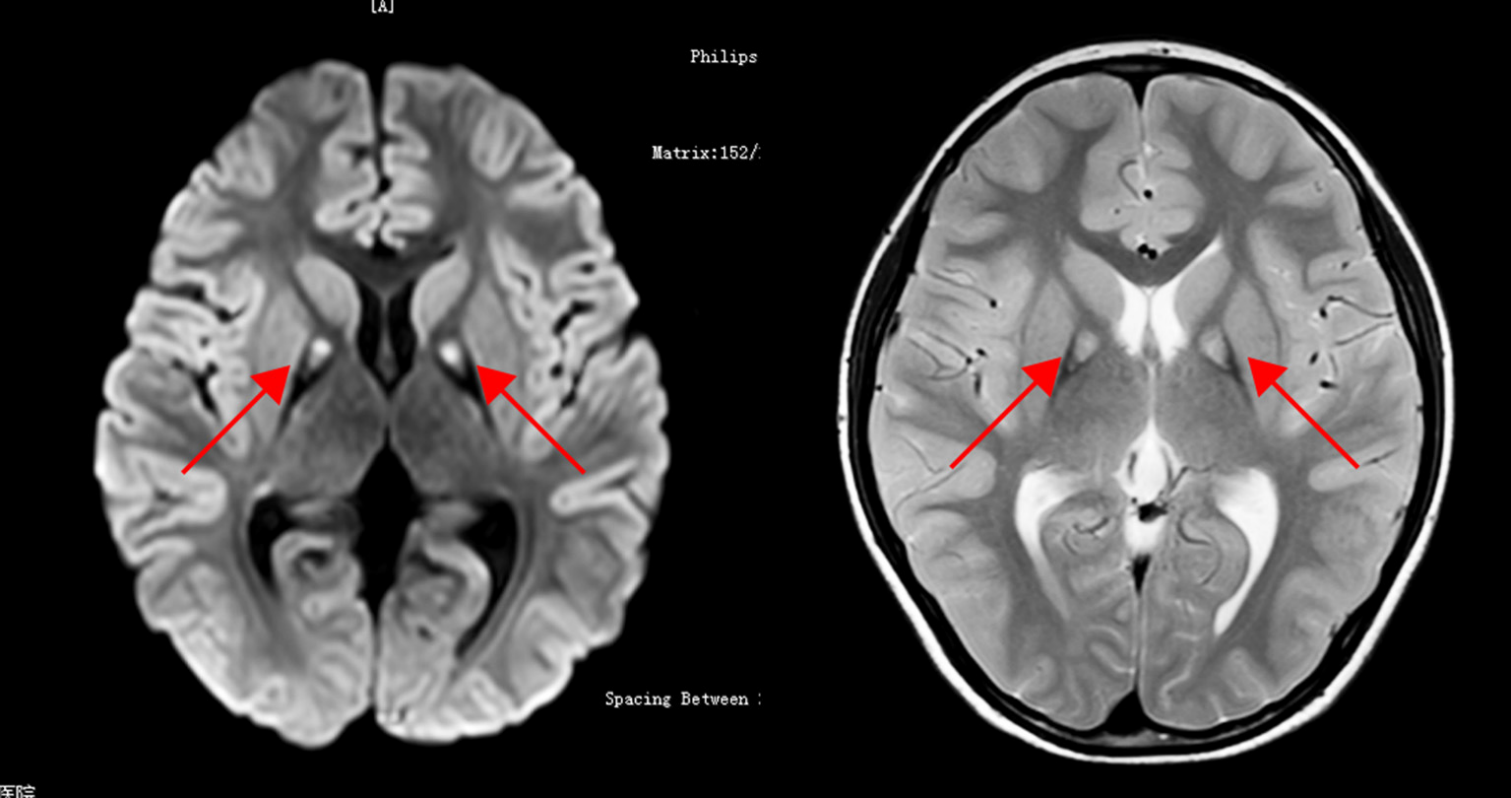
T2-weighted brain MRI showing the “eye-of-the-tiger” sign.
